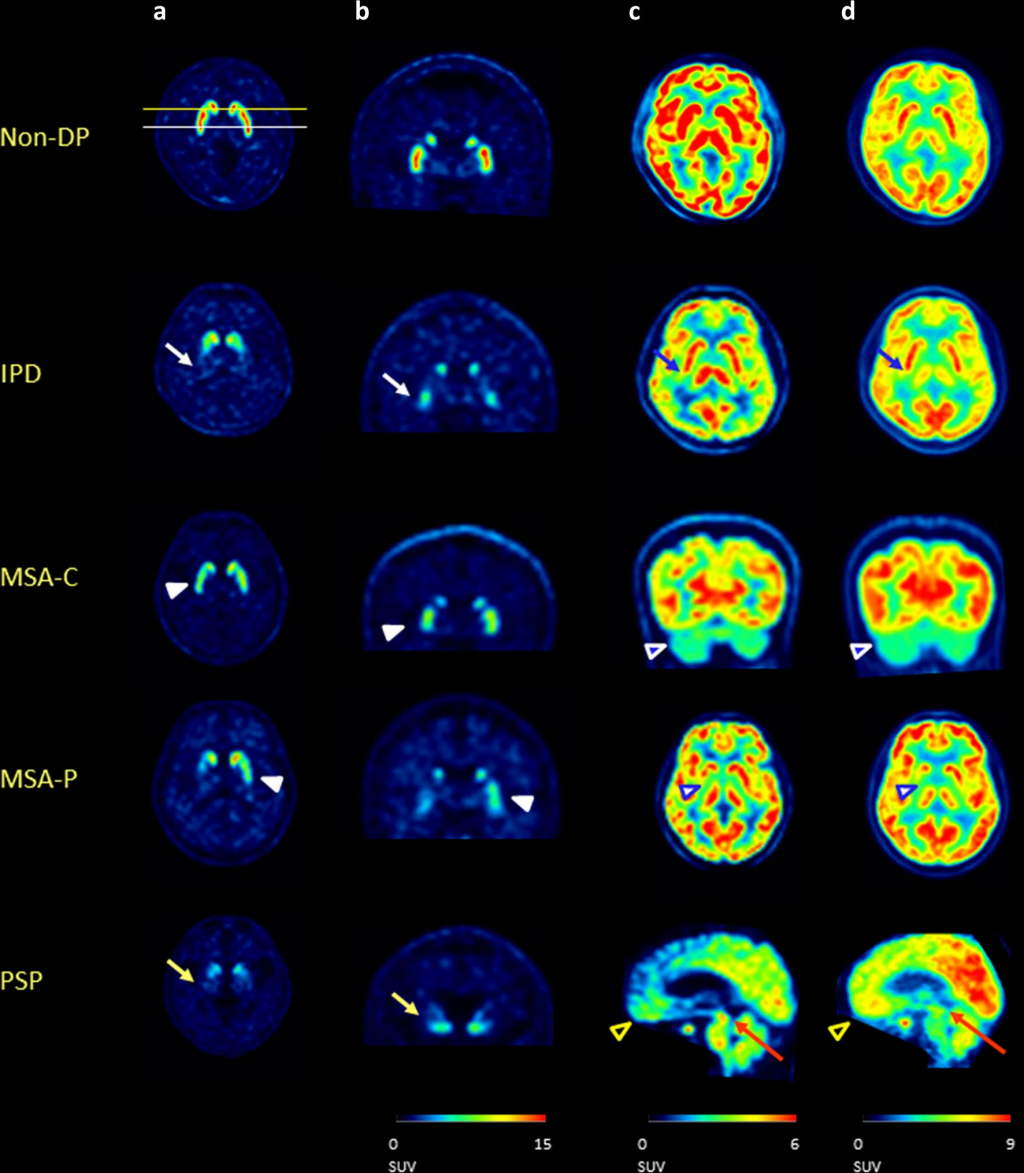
Examples of axial (a) and coronal slice (b) of delayed phase ^18^F-FP-CIT PET, early phase ^18^F-FP-CIT PET (c), and ^18^F-FDG PET (d) of patients with non-degenerative parkinsonism (1st row), IPD (2nd row), MSA-C (3rd row), MSA-P (4th row), and PSP (5th row). The patient with IPD (2nd row) showed decreased DAT binding of the bilateral dorsal posterior putamen, with relative sparing of the ventral putamen (white arrow) on delayed phase ^18^F-FP-CIT PET, and normal striatal metabolism or hypermetabolism in the bilateral dorsolateral putamen on early phase ^18^F-FP-CIT PET and ^18^F-FDG -PET (blue arrow). The patient with MSA-C and MSA-P (3rd and 4th rows), shows DAT loss not only in the posterior putamen, but also in the ventral putamen (white arrow head) on delayed phase ^18^F-FP-CIT PET. On early phase ^18^F-FP-CIT PET and ^18^F-FDG -PET, MSA showed hypometabolism in the cerebellar cortex (blue arrow head with white outline) and/or hypometabolism in the basal ganglia (blue arrow head with white outline. The patient with PSP showed extensive DAT loss on both the putamen and caudate nuclei on delayed phase ^18^F-FP-CIT PET (yellow arrow), which was characterized by hypometabolism in the medial frontal cortices (black arrow head with yellow outline) and midbrain (red arrow) on early phase ^18^F-FP-CIT PET and ^18^F-FDG PET. All PET images were processed using PMOD 4.0 (PMOD Technologies Ltd., Zurich, Switzerland).
